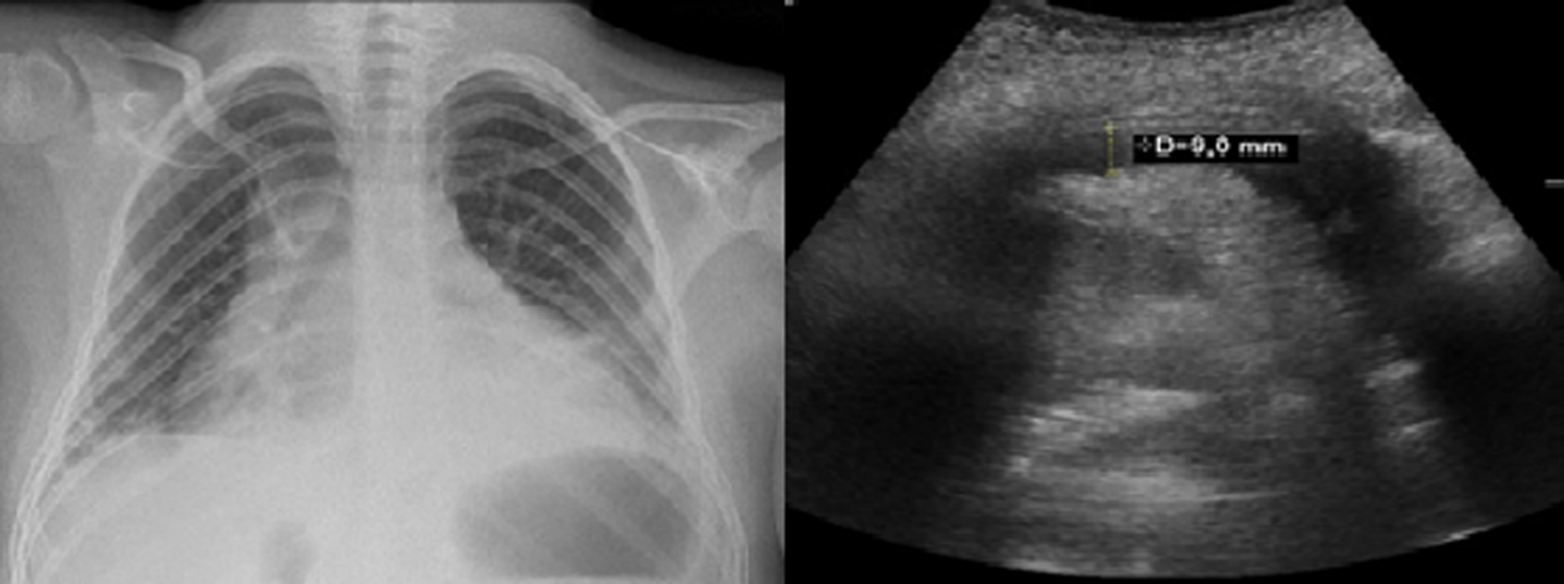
Pericardial effusion. Left: Thorax radiography showing cardiomegaly. Right: echocardiogram of patient with pericardial effusion of 9 mm.
